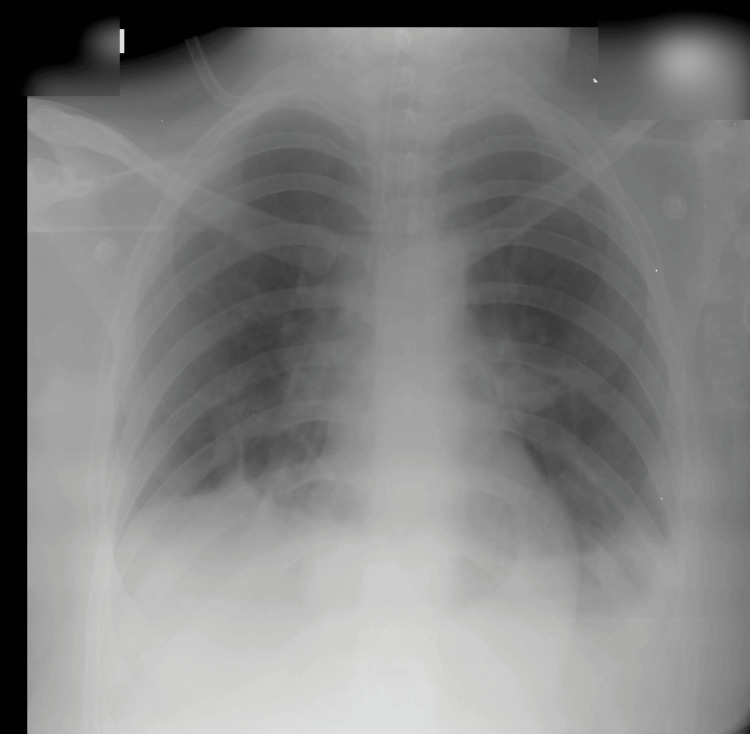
Initial portable chest radiograph on admission showing diffuse bilateral air-space opacities without pneumothorax
